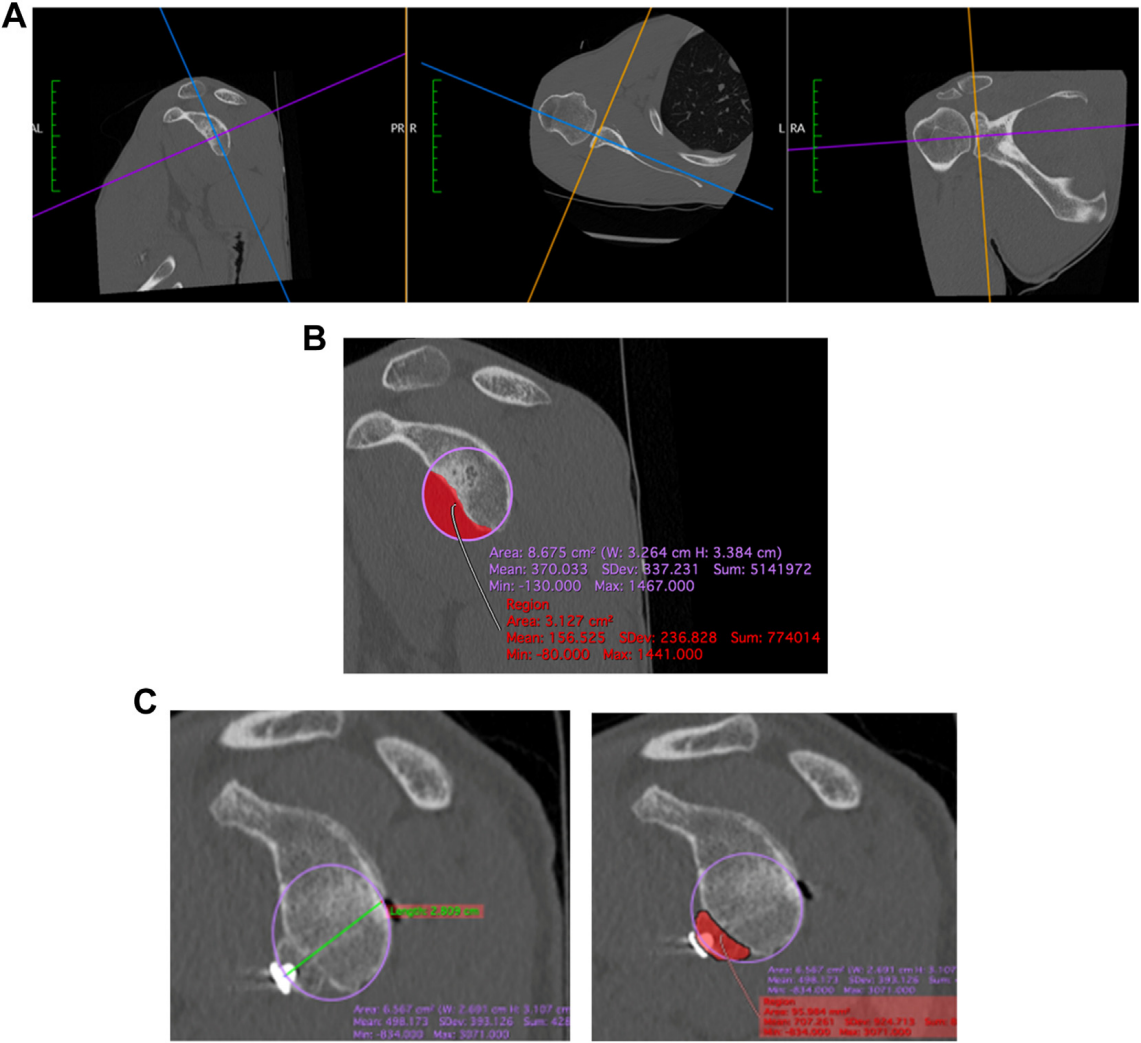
Measurement of the glenoid bone loss by the PICO method in a reformatted CT-scan. (A) The CT-scan is reformatted in the plane of the glenoid surface on the axial and coronal sections, (B) Measurement of glenoid bone loss, (C) Measurement of surface addition by the bone block. *CT*, computed tomography.
